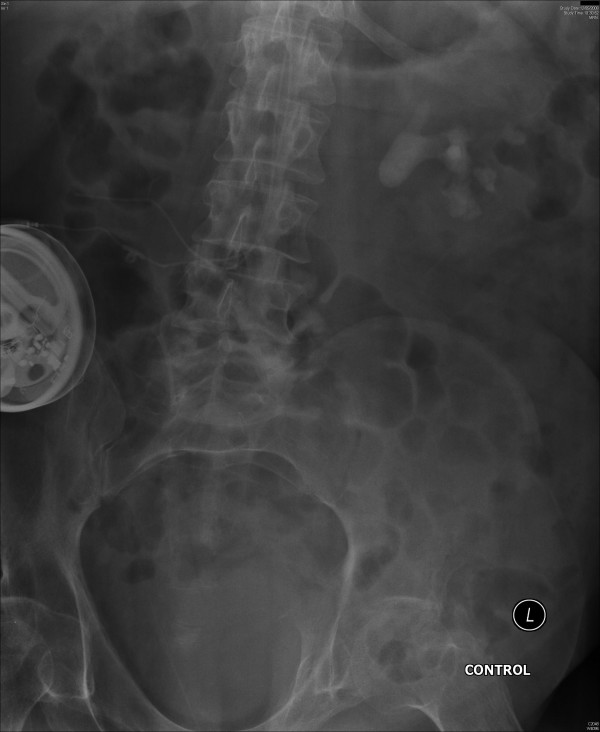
X-ray of kidneys (12 May 2008) showed stag horn calculus in left kidney.
